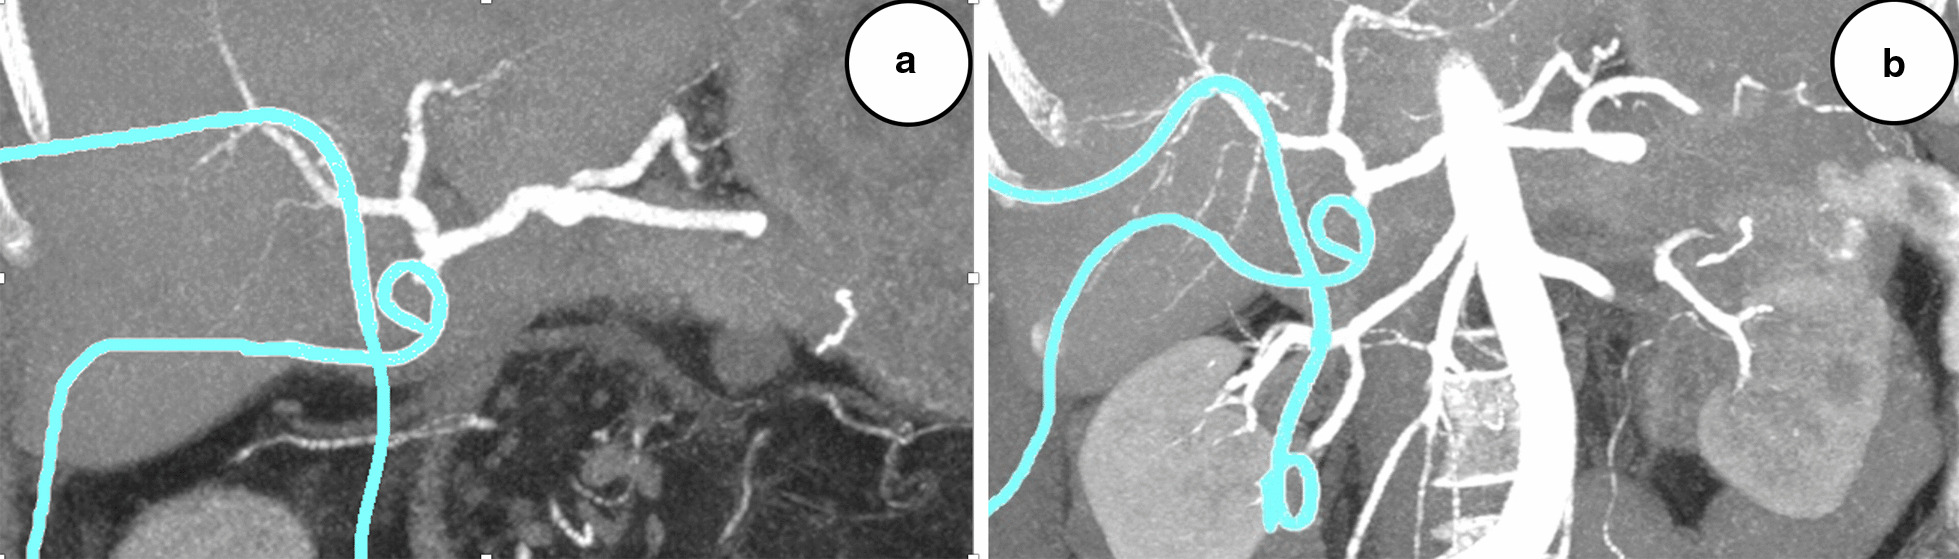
a Computed tomography angiography, coronal section of the upper abdomen, postoperative day 26, showing the celiac trunk and all main branches (splenic, left gastric, and common hepatic arteries), as well as the gastroduodenal artery stump, the proper hepatic, right hepatic, and left hepatic arteries, with no evidence of bleeding. Pigtail drains can be seen in turquoise. b Repeat computed tomography angiography of the upper abdomen performed on day 28, also without evidence of bleeding from any of the above-mentioned vessels. Pigtail drains can be seen in turquoise
